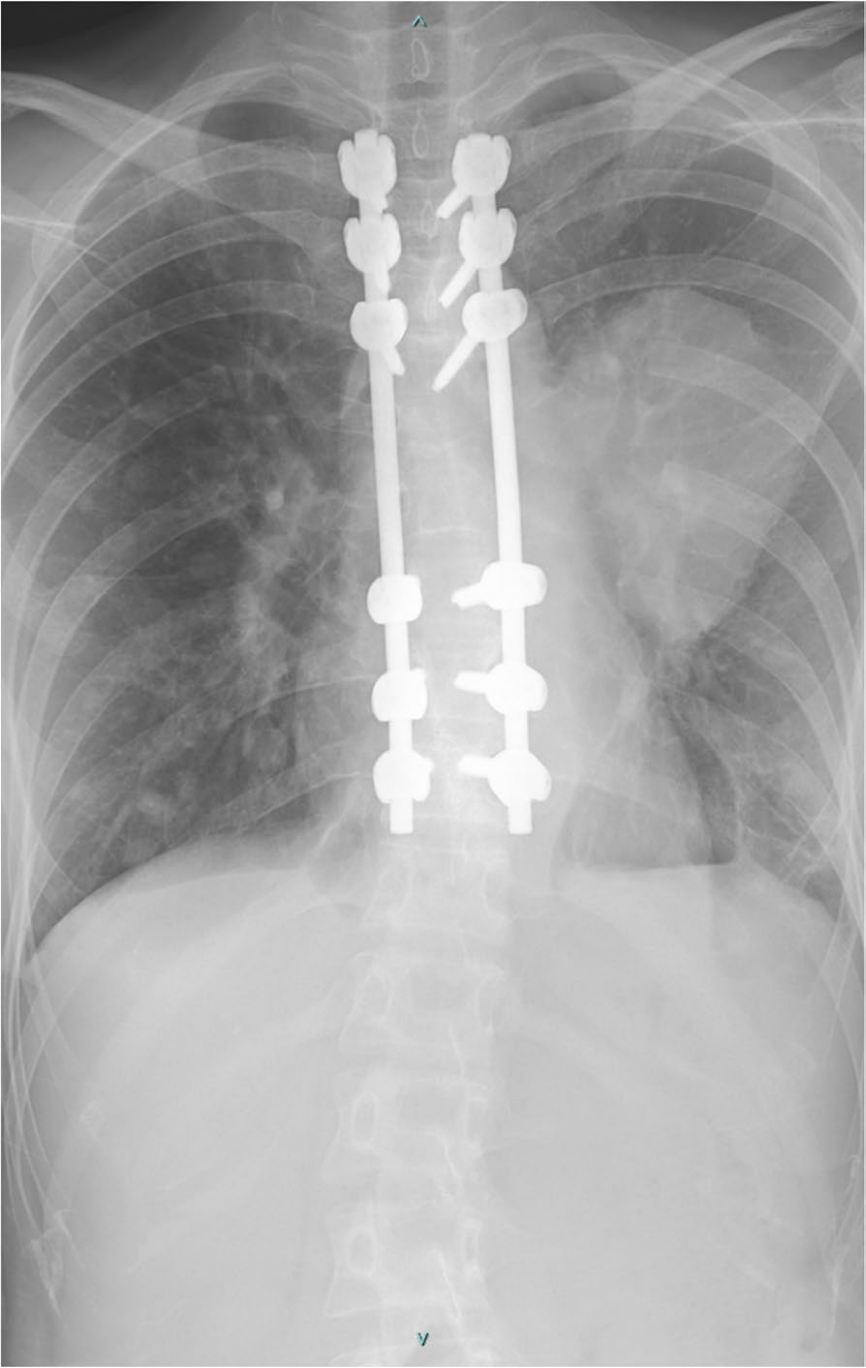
Post-operative radiograph of second operation
